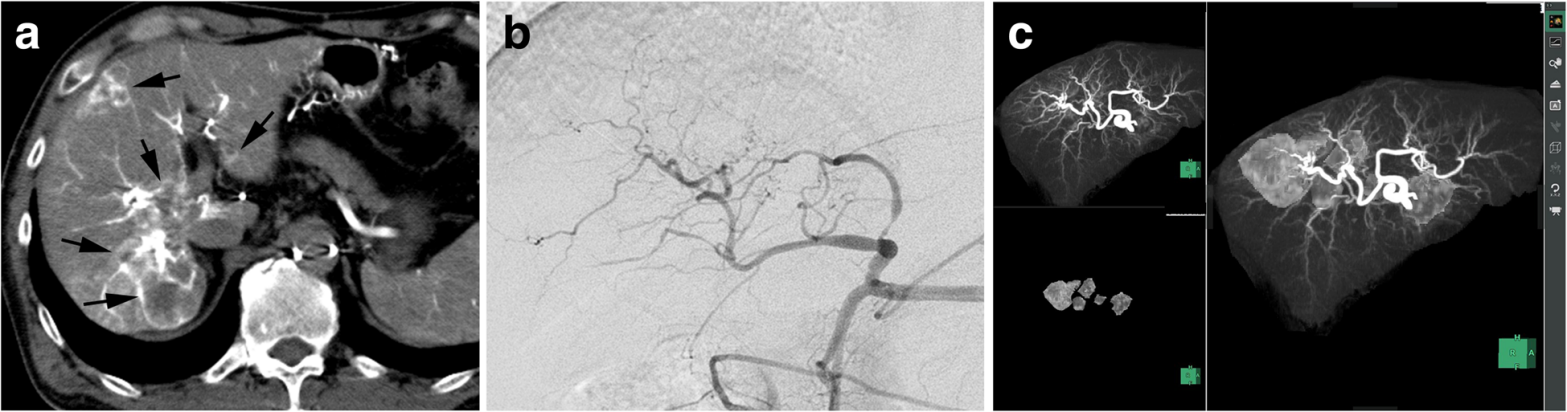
TACE navigation system by using a CT workstation. a. CT during contrast injection via the hepatic artery was obtained. Multiple metastases were seen in the right hepatic lobes (arrows). b. Hepatic angiography could not depict the tumors. c. maximum intensity projection (MIP) image of the hepatic artery and extraction of the tumors were individually created (left side). Fusion of these two images shows the tumor feeding branches of the tumors
